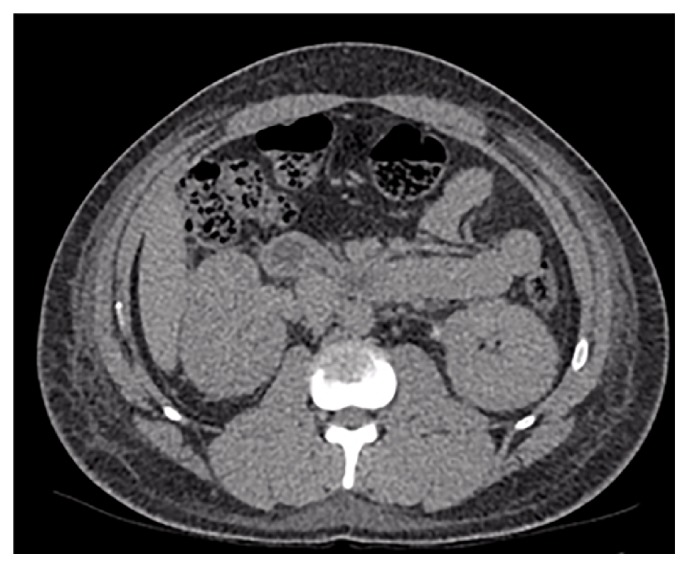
Noncontrast CT section of the abdomen, showing subcutaneous oedema.
